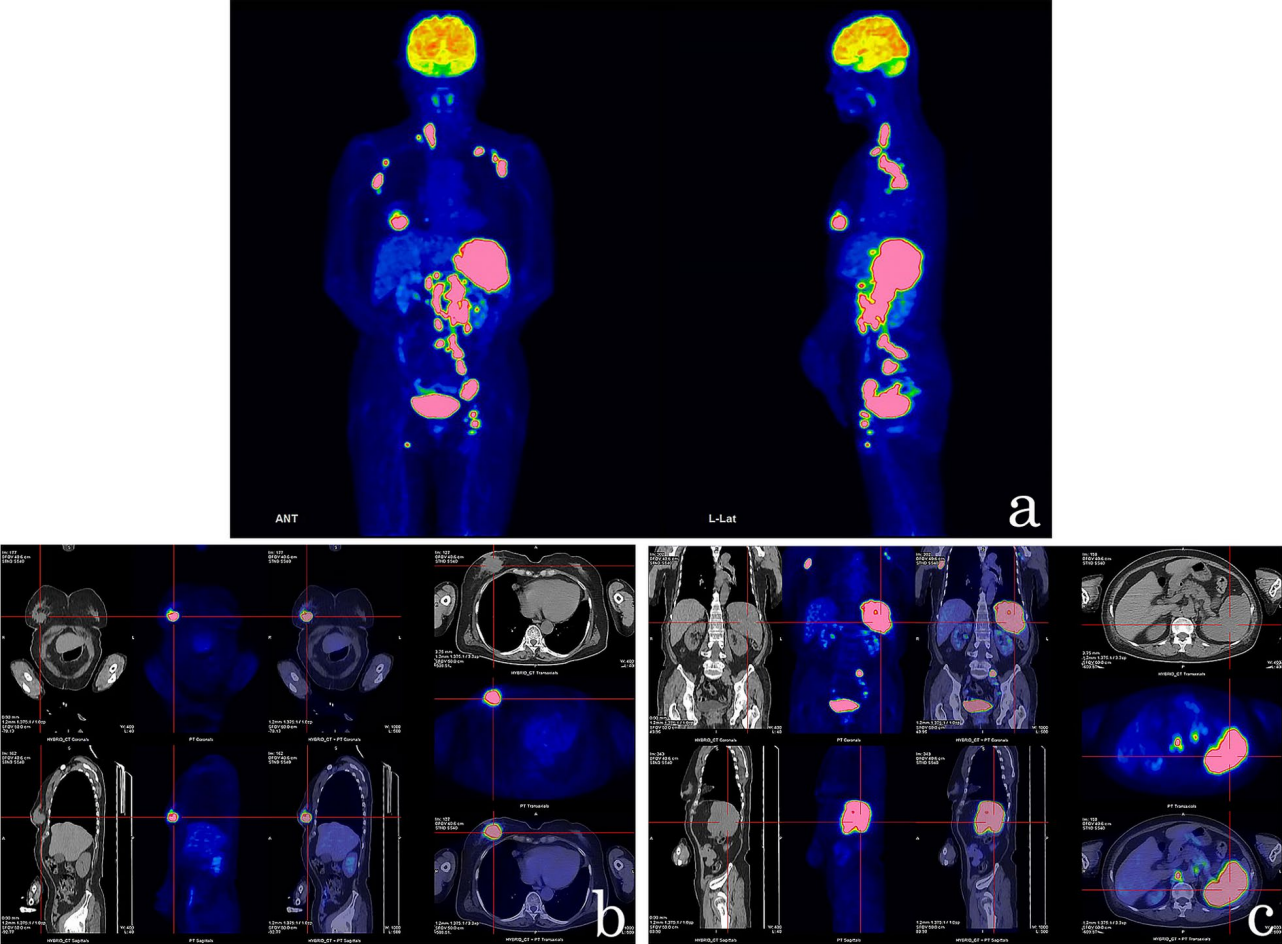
Pre-treatment PET/CT findings. PET demonstrated an accumulation of 18F fluorodeoxyglucose in the right breast, bilateral axillary, right cervical, intra-abdominal lymph nodes, spleen, and bilateral inguinal lymph nodes. The findings of the a whole body, b right breast, and c spleen are shown
